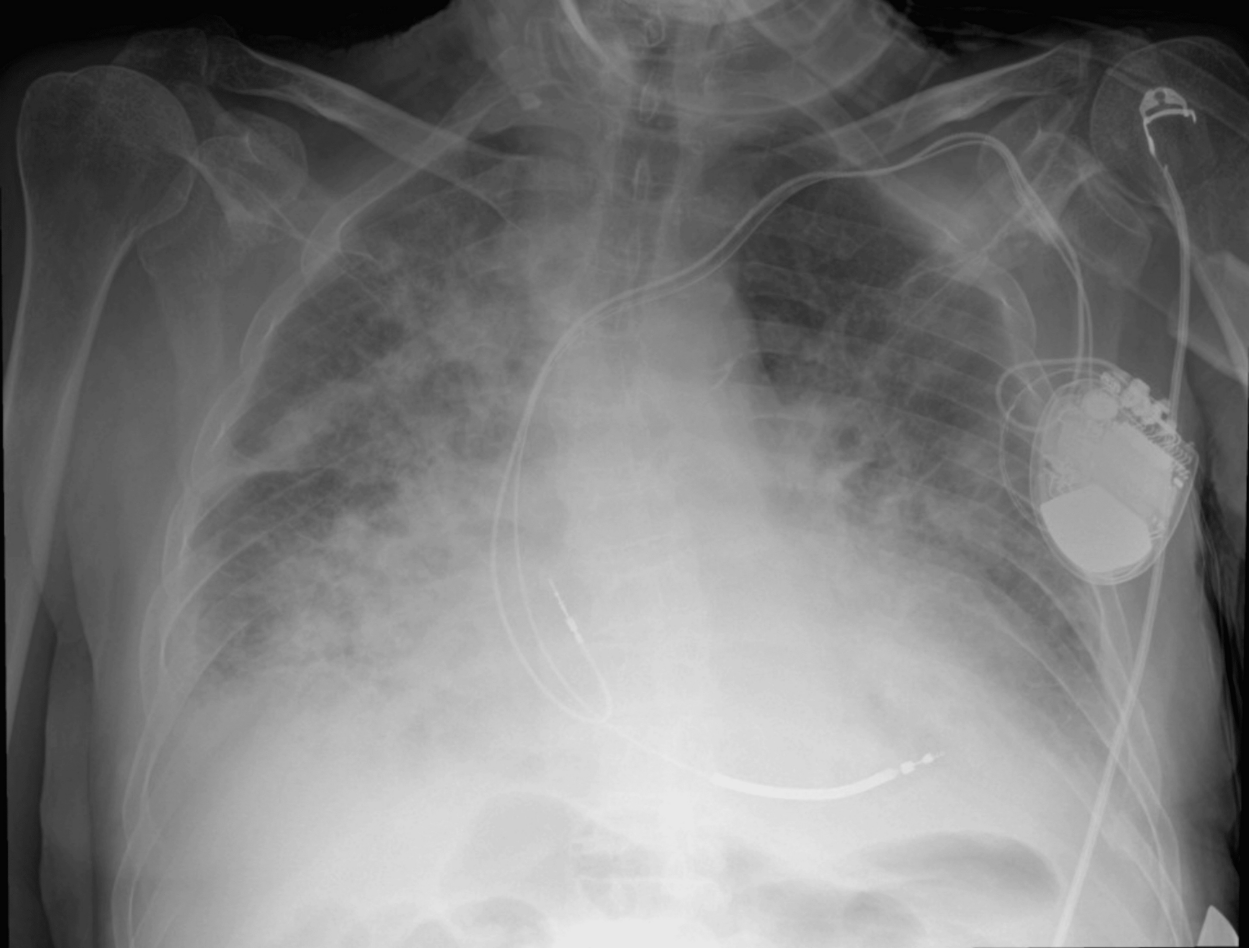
Repeat portable chest X-ray (AP view, sitting position) demonstrating bilateral perihilar opacities consistent with acute pulmonary oedema
